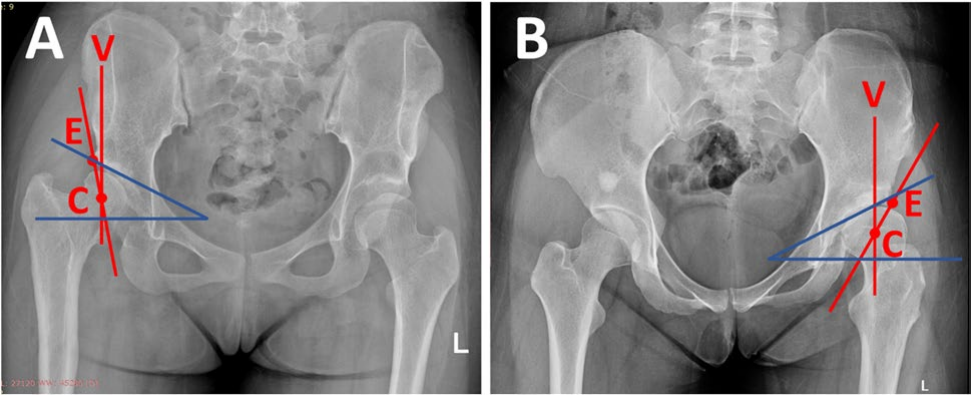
Anteroposterior pelvic radiographs of both study participants. Point C indicates the center of the femoral head, whereas point E denotes the lateral acetabular edge. The line CV represents a vertical reference through the center of the femoral head. The angle between lines CE and CV constitutes the lateral center-edge angle (LCEA). The oblique blue line connects the acetabular margins (medial to lateral), and the horizontal blue line serves as the reference plane. The angle formed between these blue lines represents the Tönnis angle (TA). A Patient 1, (B) Patient 2
